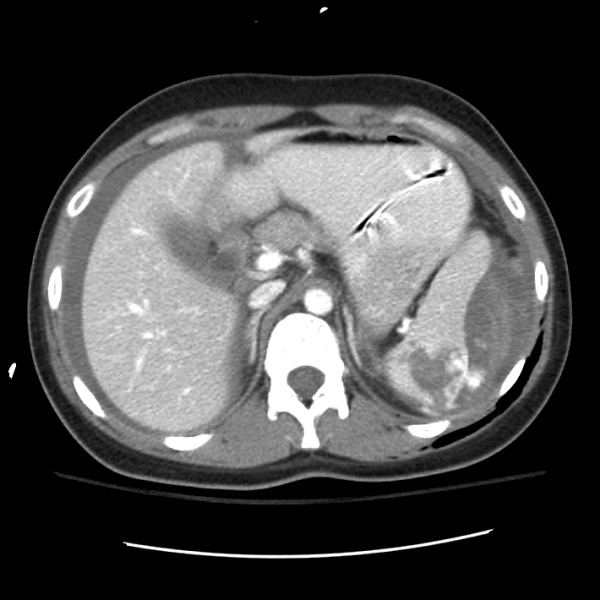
Abdominal CT scan of same patient revealing active contrast extravasation at 08:13 am.
